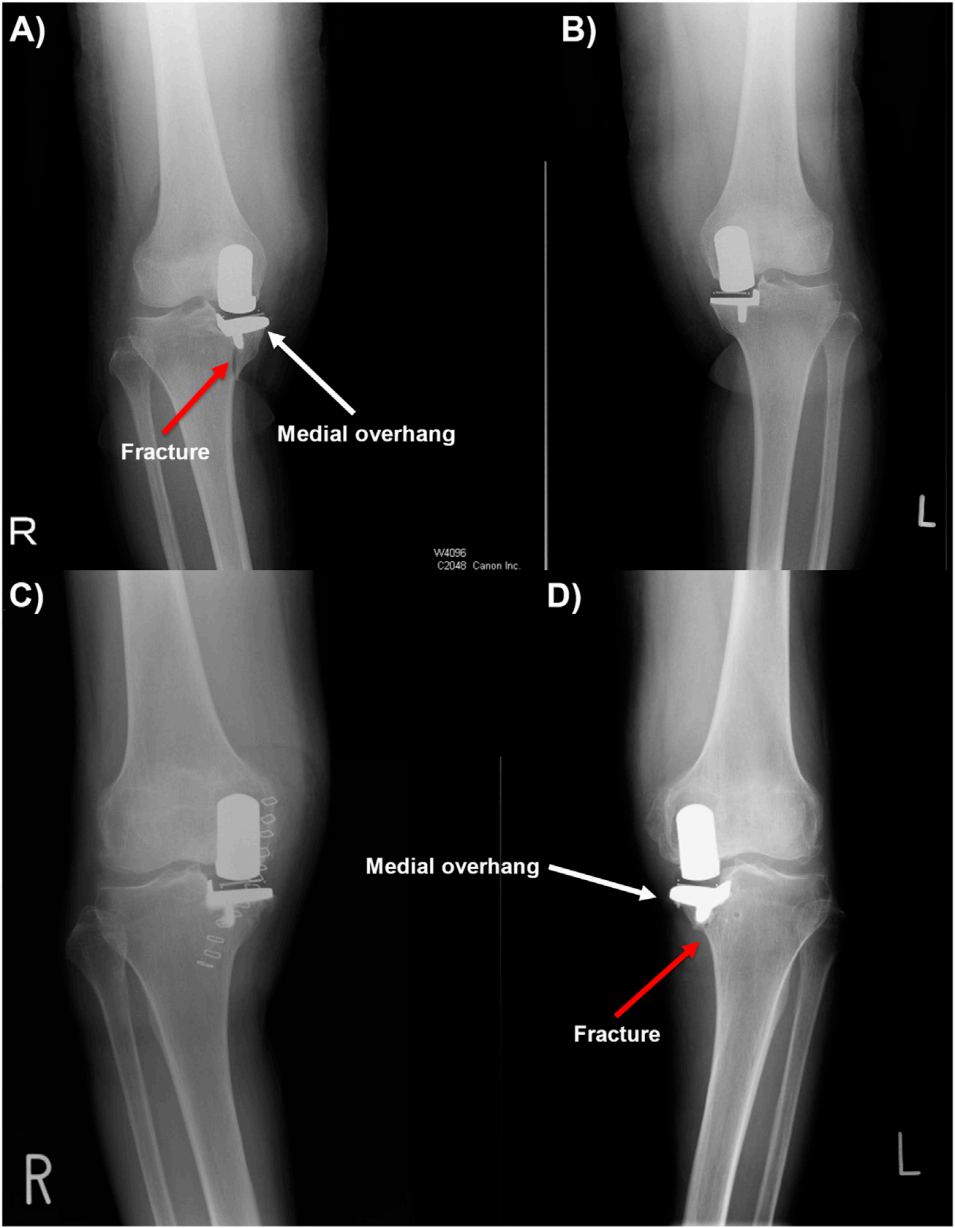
(A, B) Post-operative anteroposterior radiographs of patient 1, showing periprosthetic fracture in the right tibia. (C, D) Post-operative anteroposterior radiographs of patient 2, showing periprosthetic fracture in the left tibia with tibial component medial overhang.
